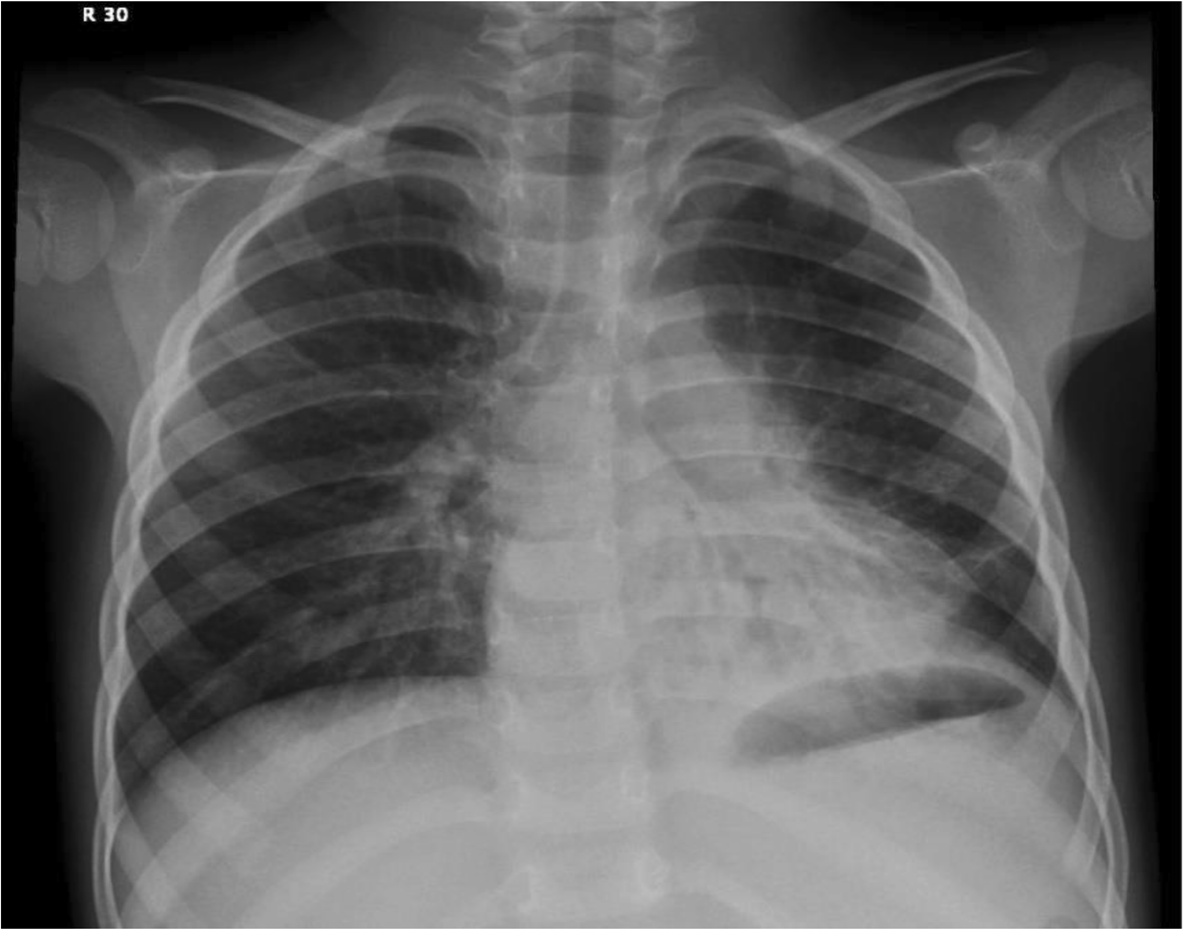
Chest X-ray before the bronchoscopy showing a significant lower lobe consolidation with Bronchiectasis in in the same lobe
